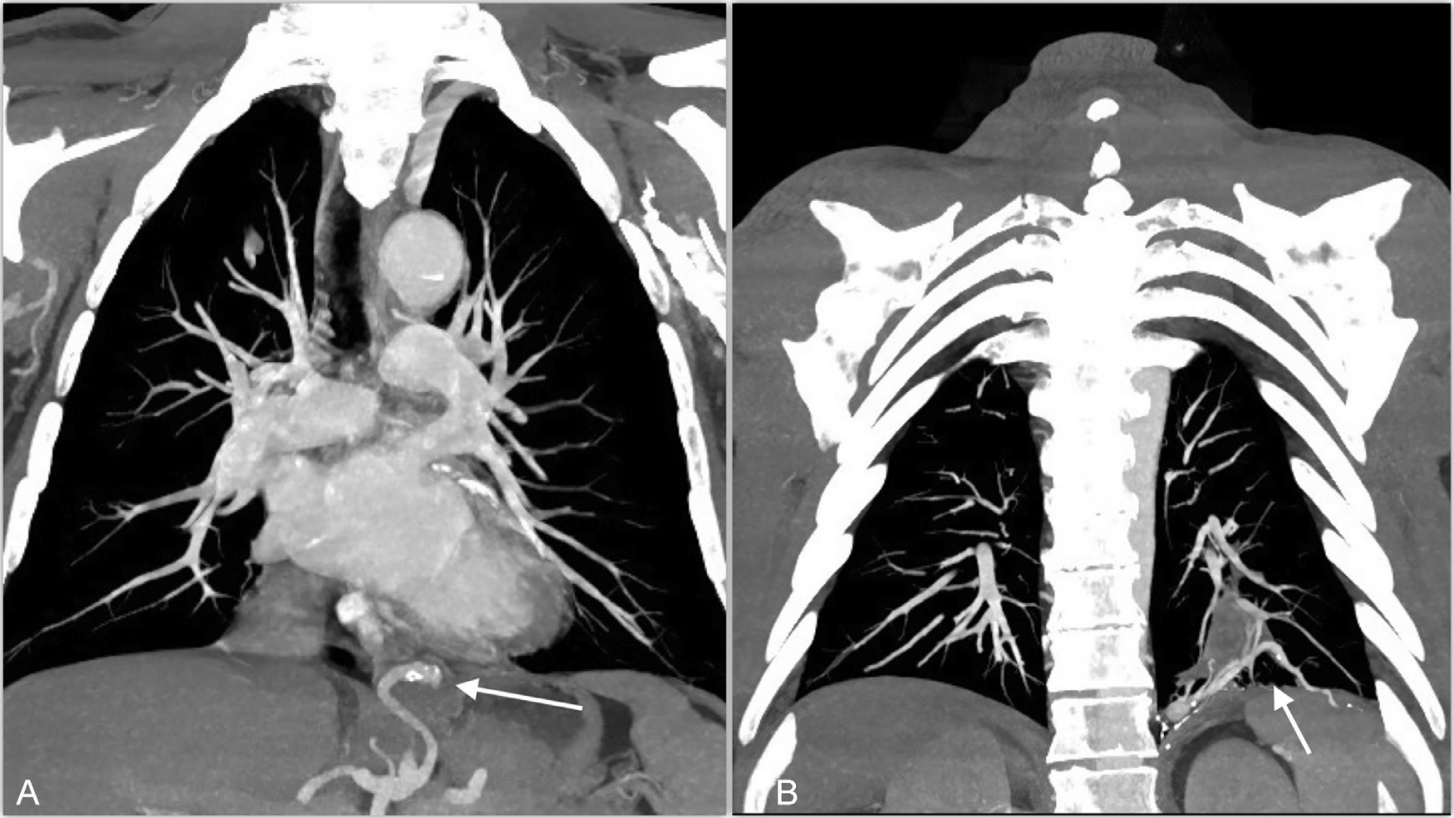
(A,B): coronal view MIP CT showed the arterial aberrant branch originating from the celiac trunk, passing through the diaphragm and suppling the left lower lobe (arrows).
